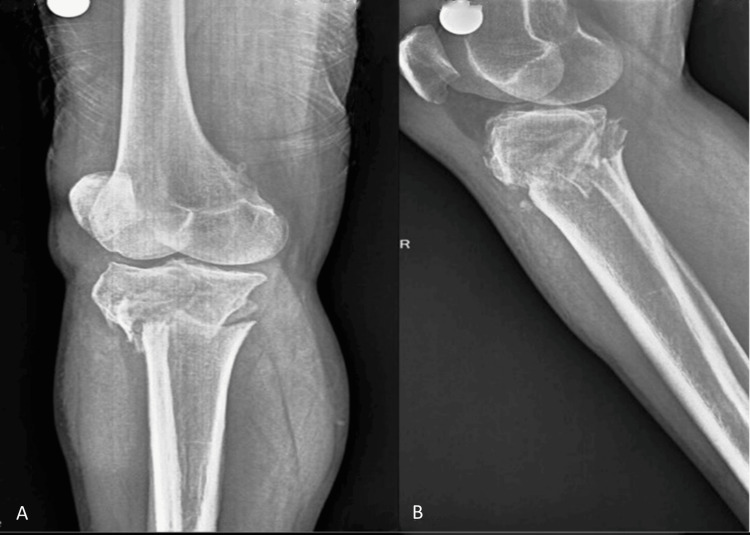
Preoperative radiographs of the right knee joint. (A) Anteroposterior and (B) lateral radiographs show comminuted intra-articular fracture of the medial and lateral tibial plateau with metadiaphyseal fracture.
